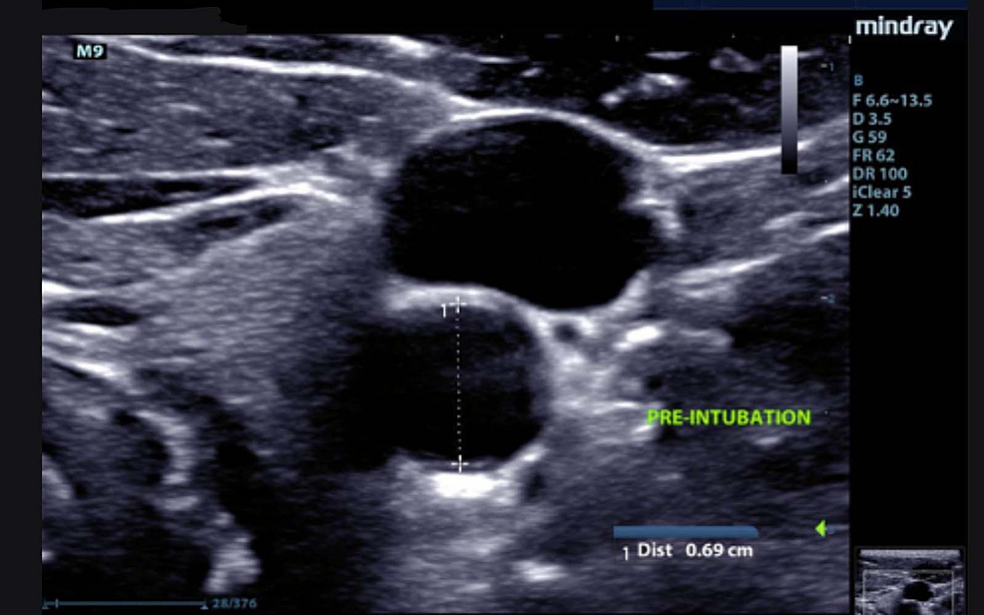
Carotid Artery Diameter The carotid artery vessel diameter was measured in transverse at the height of the thyroid gland or 3 cm below the carotid bulb if no thyroid gland were present. Pulse wave Doppler measurements were obtained at this same location. The diameter was transferred to the longitudinal image to allow the ultrasound machine to calculate the area and flow volume.
